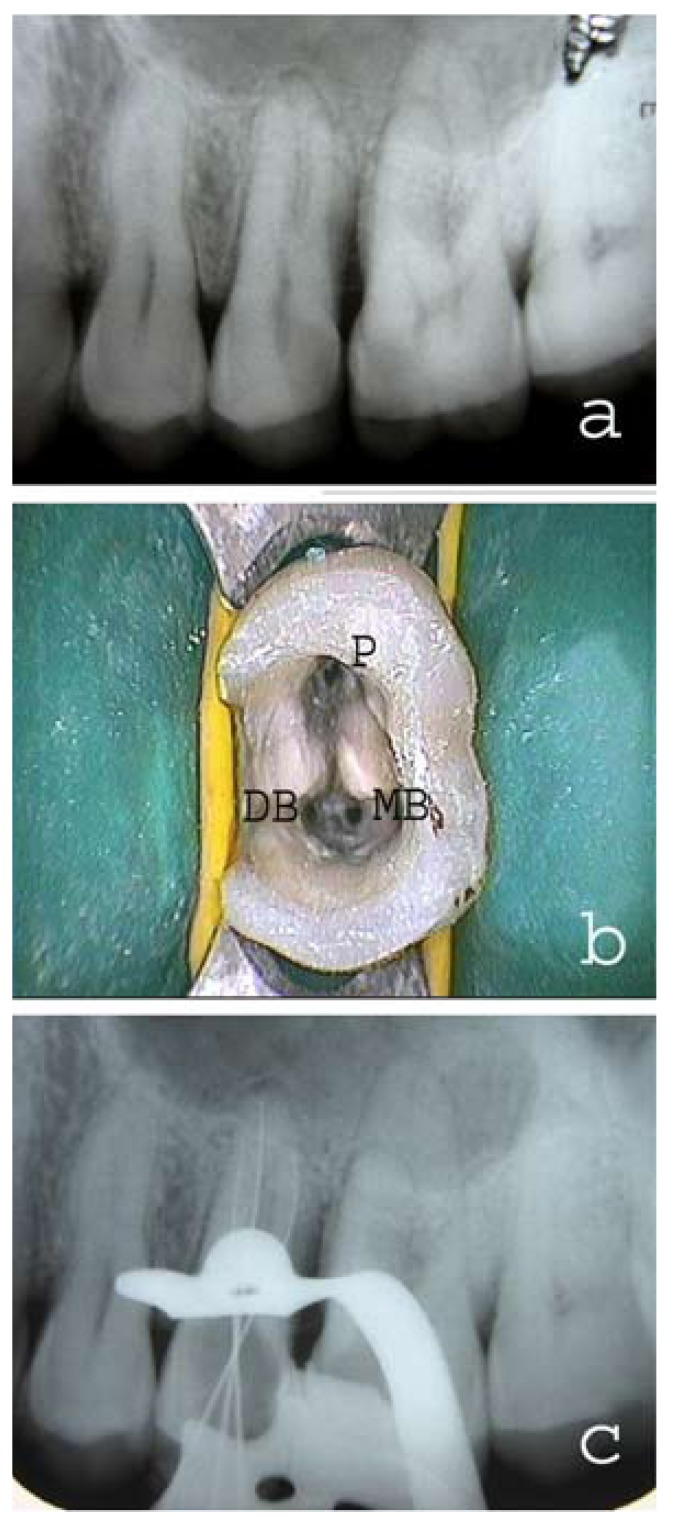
(a) Preoperative radiograph showing the presence of three separate roots in left maxillary second premolar. (b) The pulpal floor as seen after access opening. The three root canal orifices were located in the same way as in maxillary molar (P-Palatal, MB-Mesiobuccal, DB-Distobuccal). (c) Radiographic confirmation of working length.
